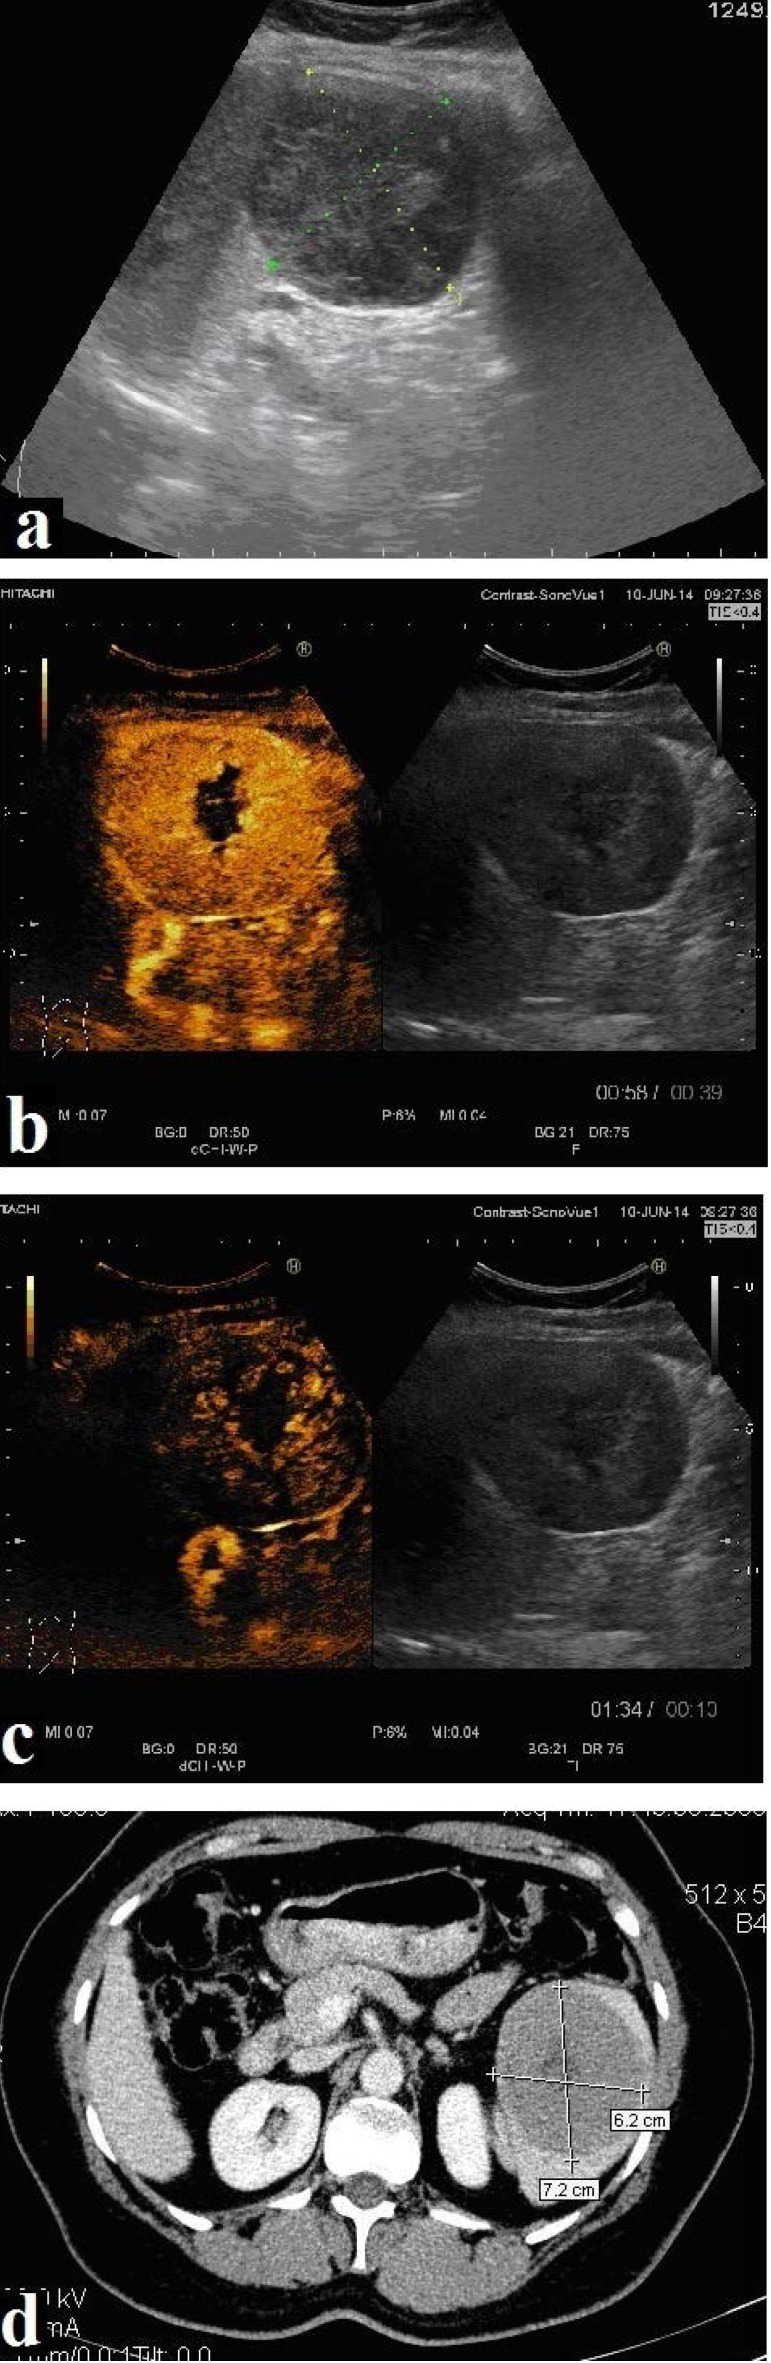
Abdominal ultrasound: splenic mass 7.4/ 6.8 cm, round-oval in diameter, located at the inferior pole of spleen, with an intense inhomogeneous structure (Fig. 1a). Contrast enhanced ultrasonography in the arterial phase shows a rapid uptake of the contrast agent in the splenic mass, 10 seconds after injection, with a central area of necrosis (Fig. 1b). Rapid and complete washout is revealed in the late phase (Fig. 1c). Computer tomography shows heterogeneous mass located at the lower pole of spleen spontaneously isodense, moderately iodophilic, with necrotic areas and minimal extracapsular extension (Fig. 1d)
